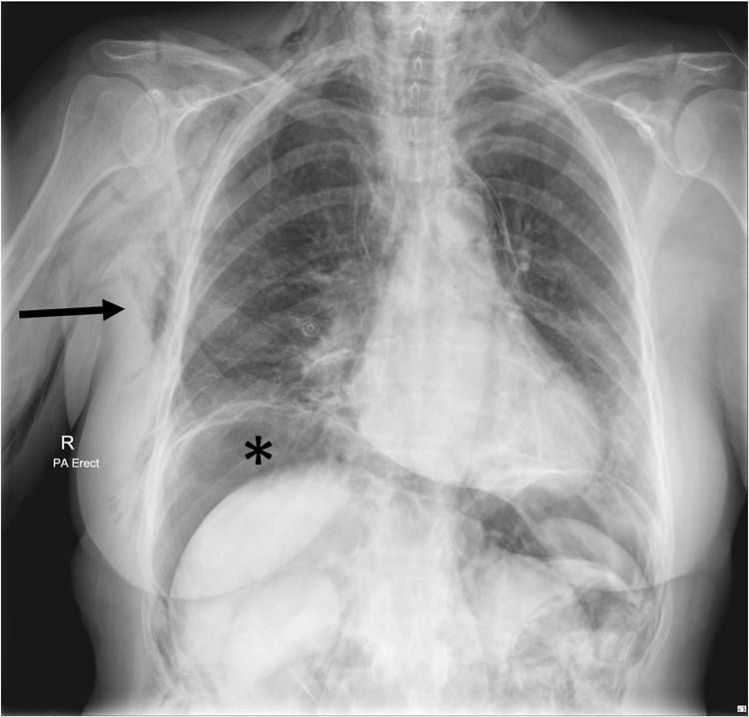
Chest X-ray Day 2 post-operatively, showing extensive subcutaneous emphysema on the right (arrow) and subdiaphragmatic free gas (*) concerning for perforated viscus.
